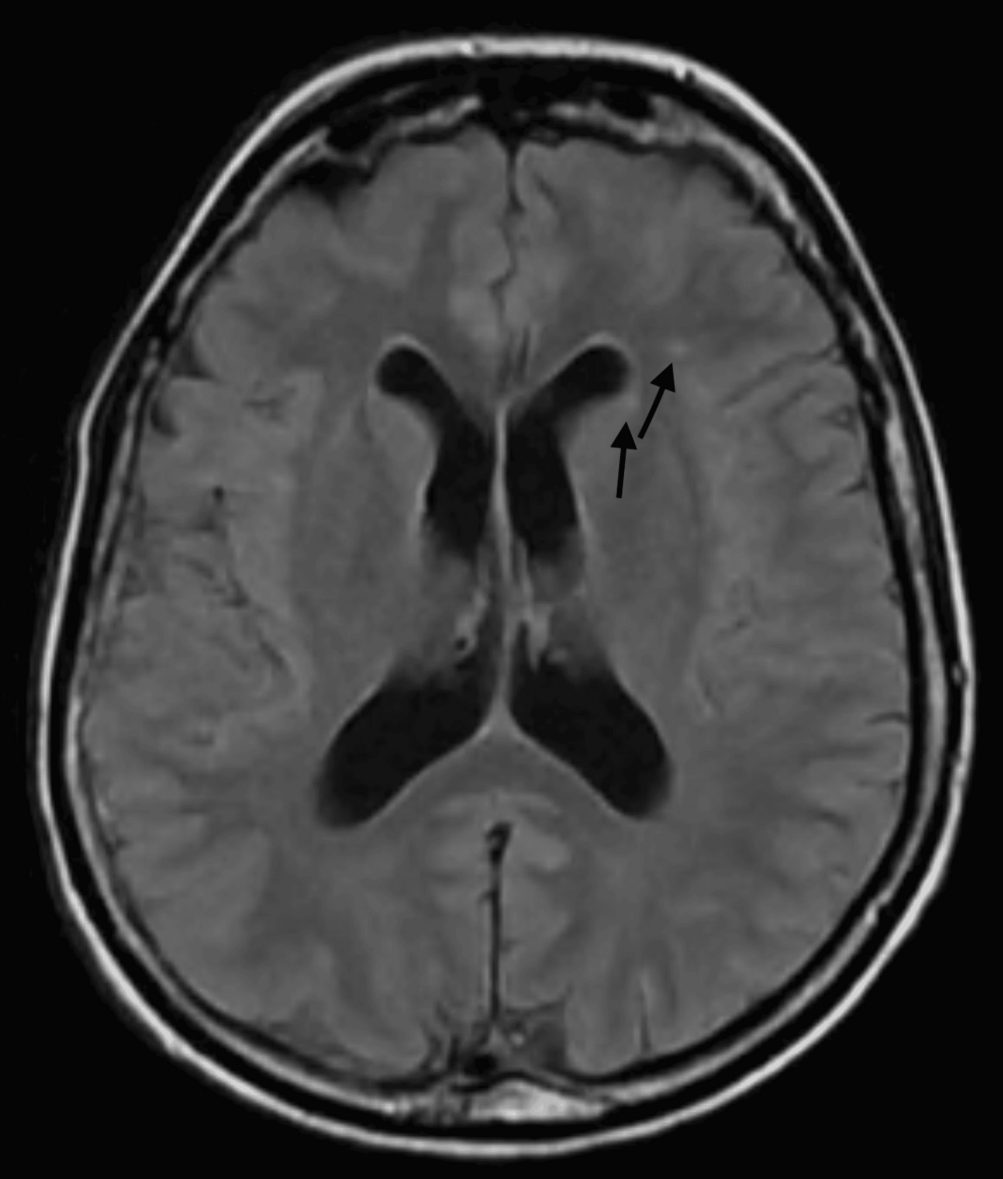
MRI brain showed incidental few punctate T2 and FLAIR subcortical white matter hyperintensities (marked by arrows). FLAIR: fluid-attenuated inversion recovery.
